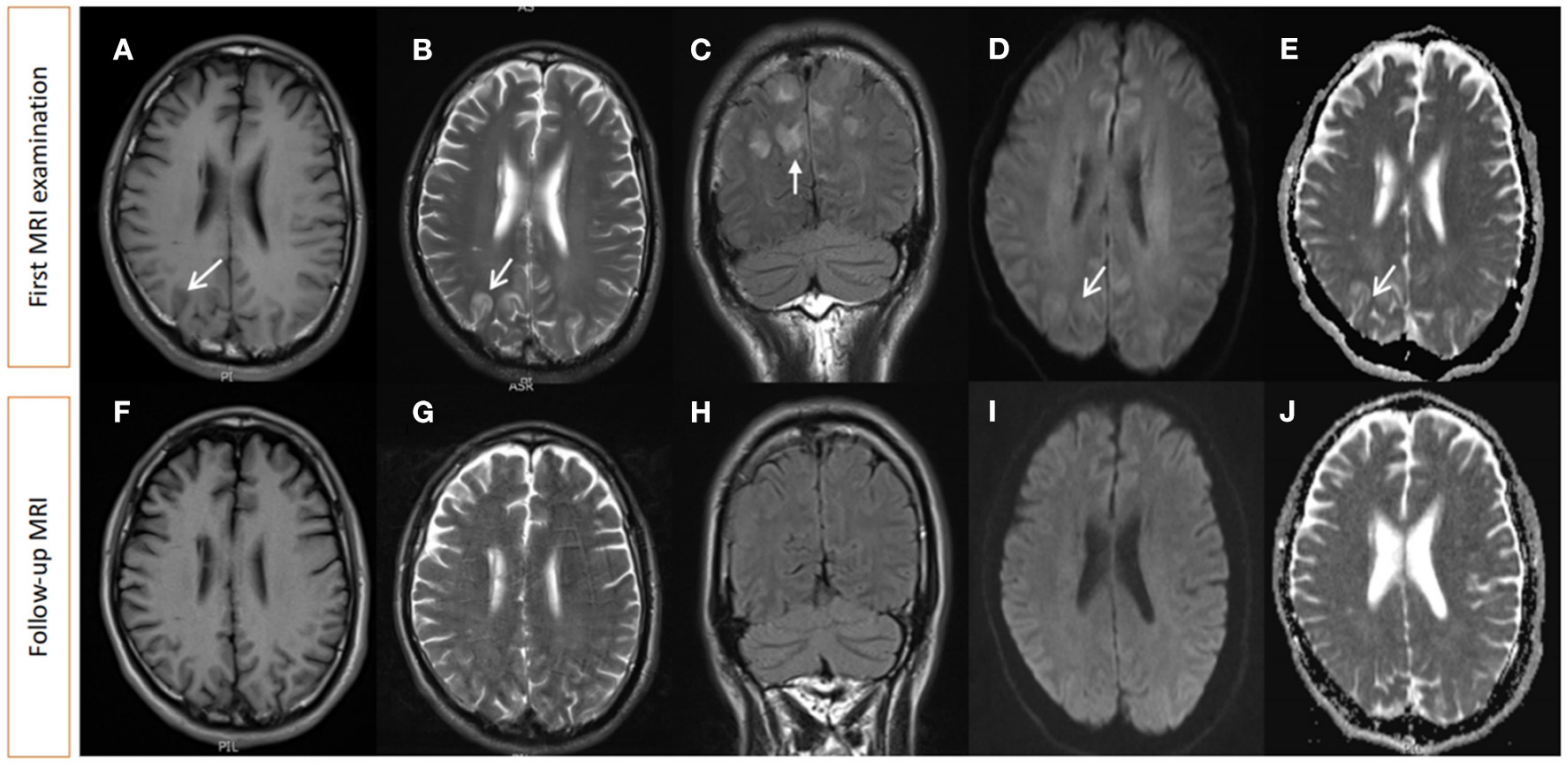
Brain MRI 19 days after symptom onset and at follow-up 3 months later. (A) T1 hypointensity and (B) T2 hyperintensity can be seen in the cortex and subcortex of the bilateral parietal lobe and the occipital lobe, with hyperintensity on T2 FLAIR (C), DWI (D), and ADC (E). Follow-up MRI shows abnormalities have disappeared (F–J). MRI, magnetic resonance imaging; FLAIR, fluid-attenuated inversion recovery; DWI, diffusion-weighted imaging; ADC, apparent diffusion coefficient.
